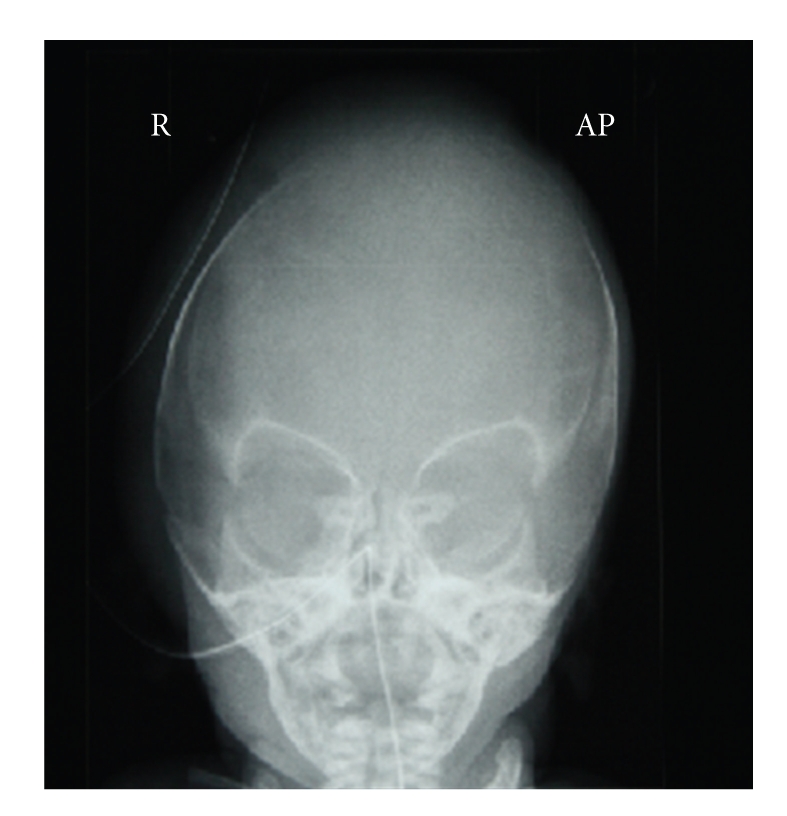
X-Ray demonstrating deviation nasal septum to right.
